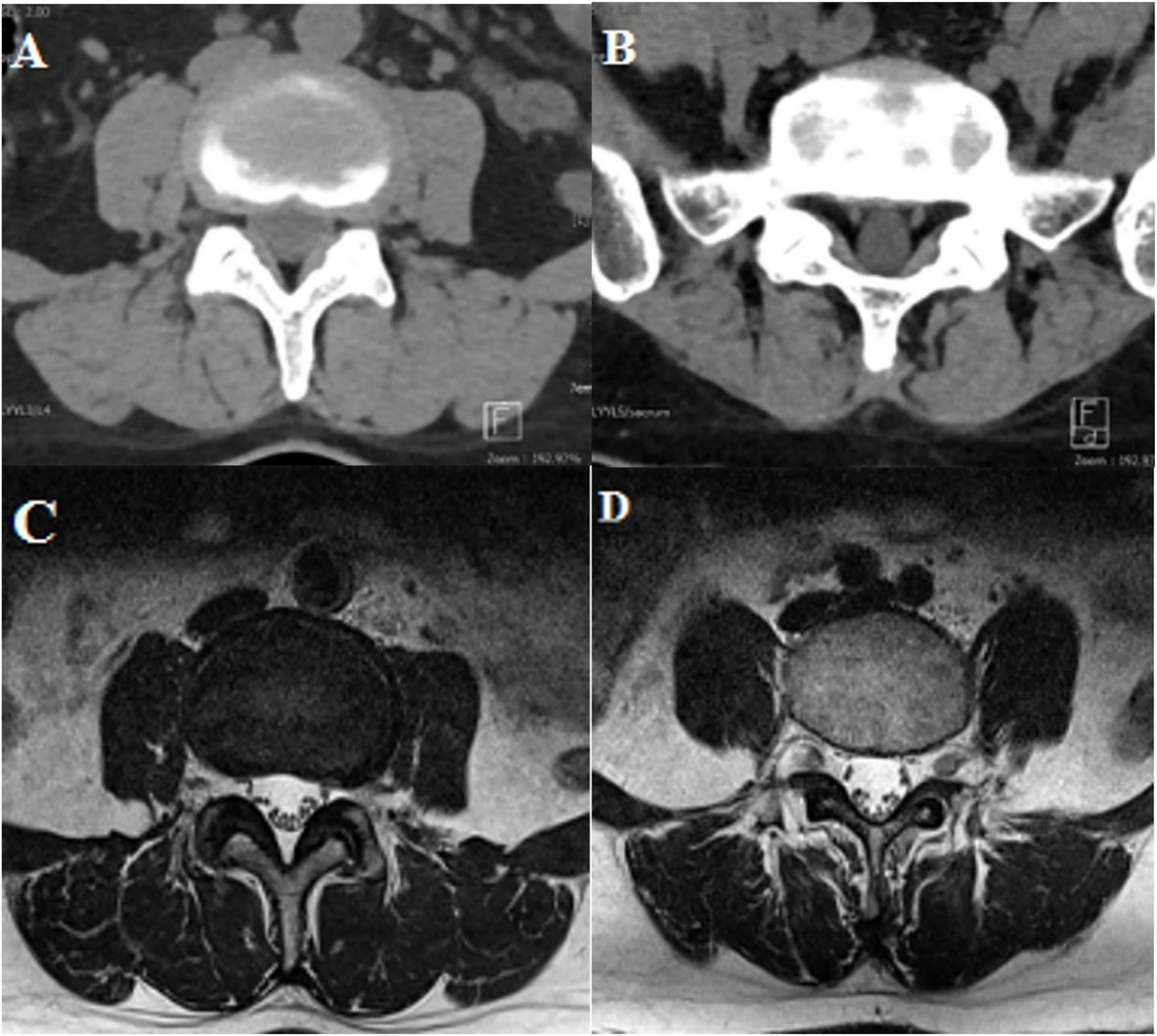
An example of Goutallier grading system on CT (a, b) and MRI (c, d) in a 49-year old woman. a grade I: low density fat is observed around the vertebral lamina. b grade II: The fat surrounding three borders of the multifidus muscle is observed, and there was atrophy change. c grade I: In the axial T2-weighted image, there is fatty streak in the paraspinal muscles and fat surrounding the lamina. d grade II: on axial T2-weighted images, the amount of fat in the muscle is increased and accompanied by atrophic change
